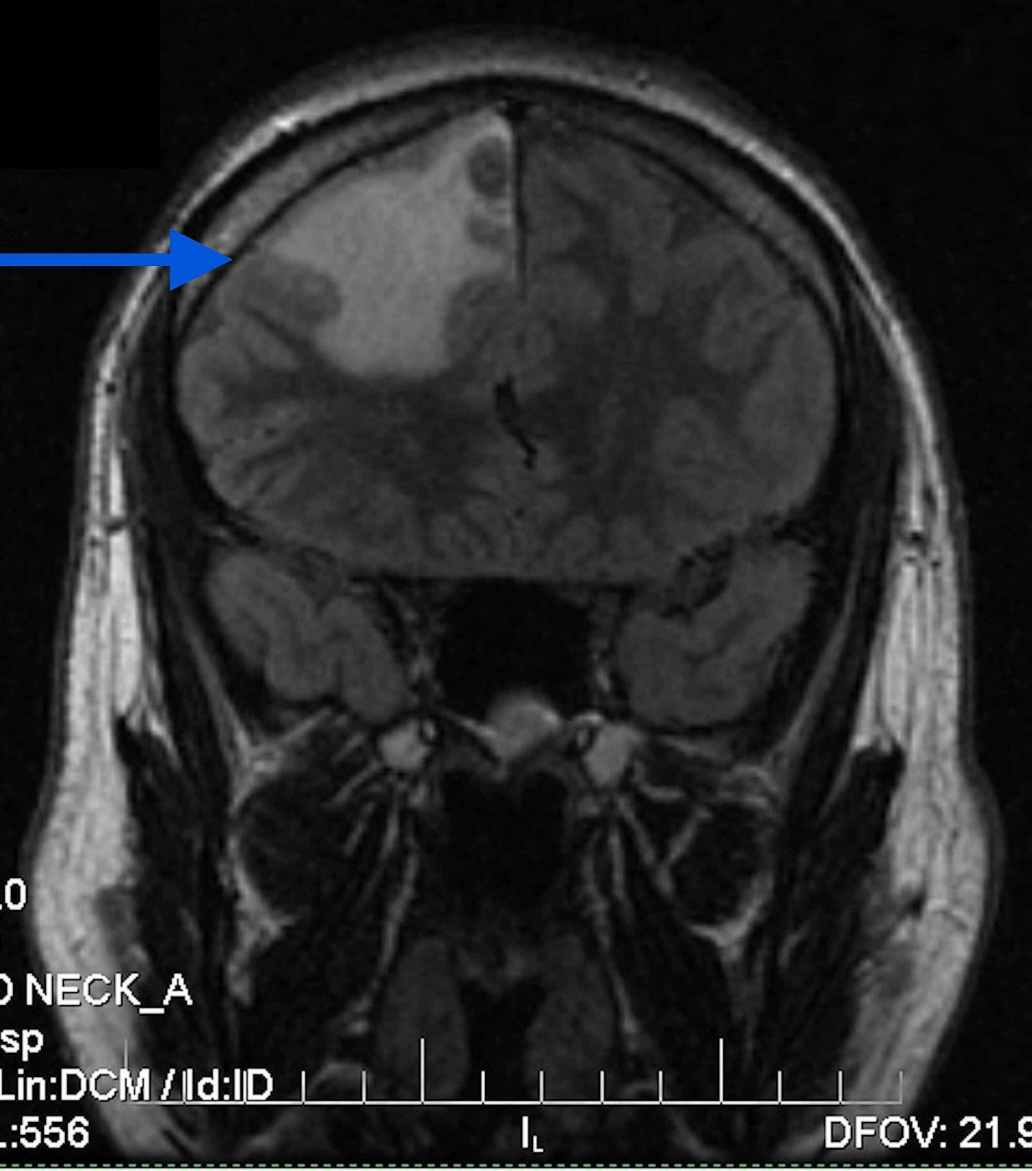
Coronal T2 FLAIR MRI showing 16×14×21 mm conglomerate ring-enhancing lesion in the paramedian region of the right frontal lobe with surrounding edema. FLAIR: fluid-attenuated inversion recovery
